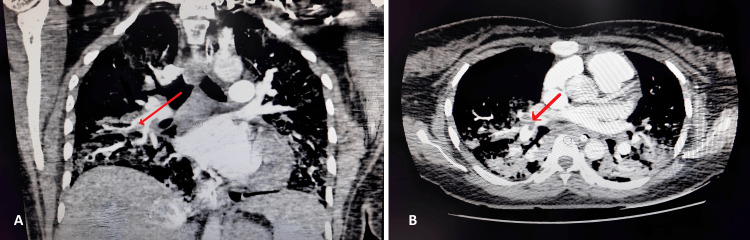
Coronal (A) and axial (B) CTPA confirmed acute thromboembolism in the lumen of the right interlobar, right middle lobar, and right lower lobar pulmonary artery. CTPA: computed tomography pulmonary angiography.
